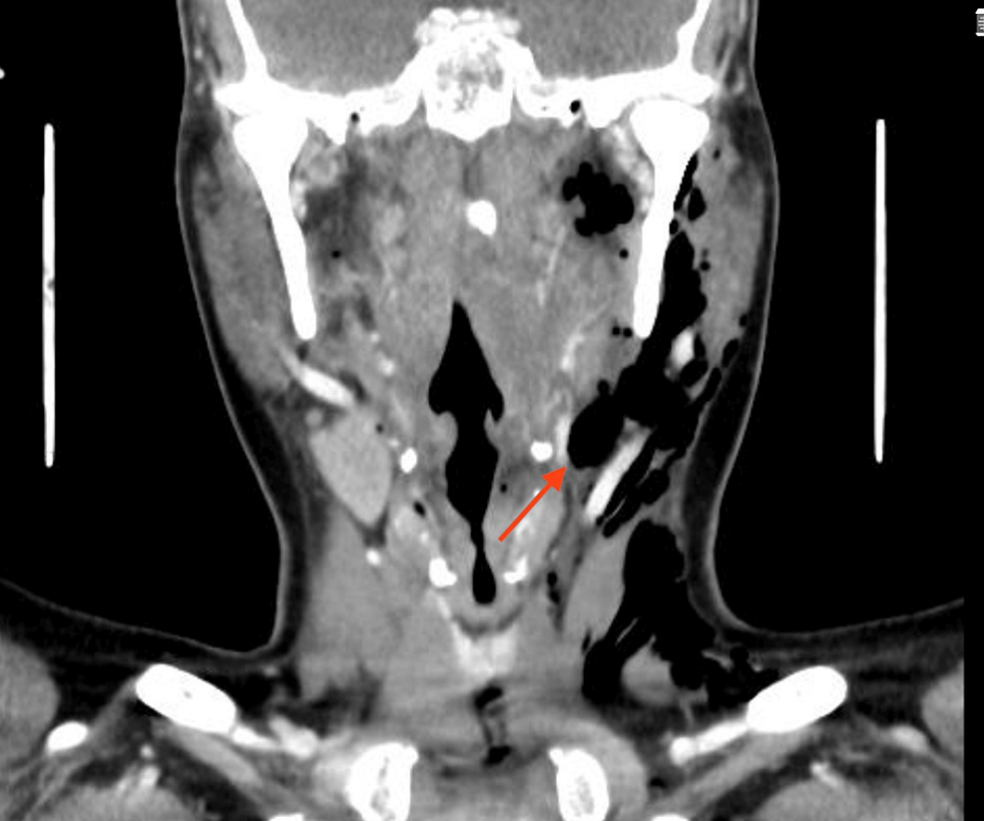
CT with contrast, coronal view The arrow pointing to air extending to parapharyngeal space into the rest of the neck spaces on the left side.
